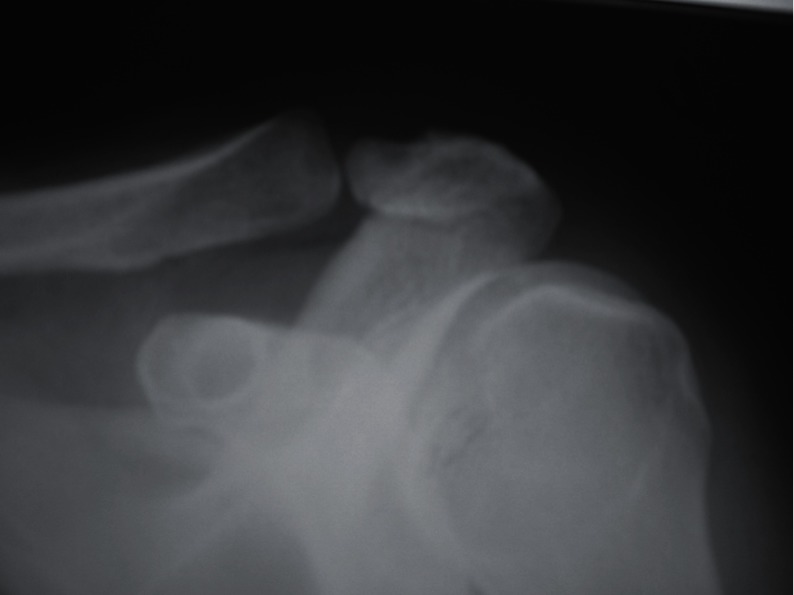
Radiograph of the Shoulder Revealed Complete Healing 14 Months Following the Treatment
